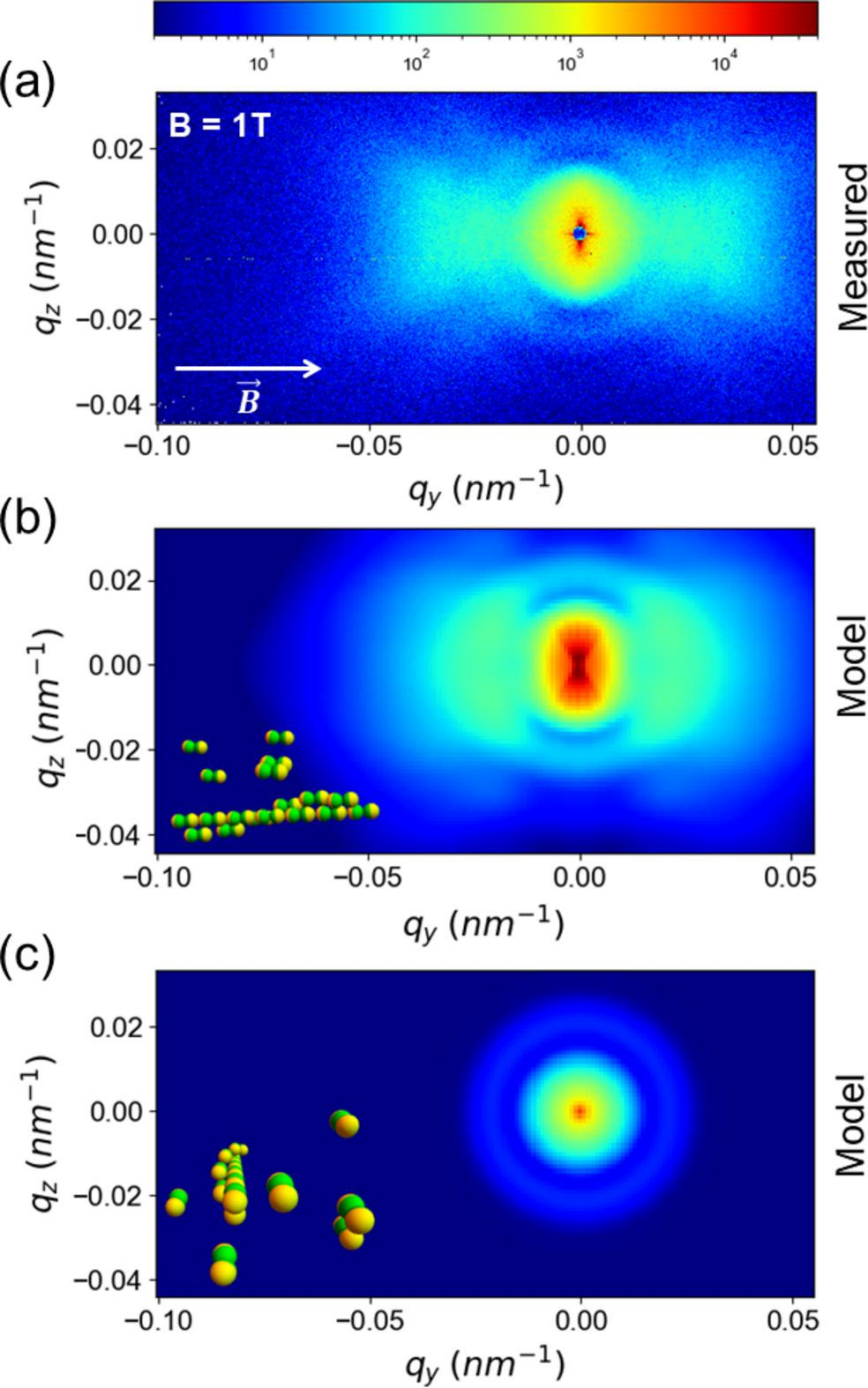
(*a*) A representative anisotropic 2D USAXS pattern from JPs for an applied B of 1 T perpendicular to the X-ray beam direction. Notice the central streak formed by the chain-like configurations of JPs. (*b*) Corresponding modeling in terms of equations (18[Disp-formula fd18]) and (23[Disp-formula fd23]) using the same structural parameters as in Fig. 4 ▸ for γ = ±30°, δ = ±30°, σ_
